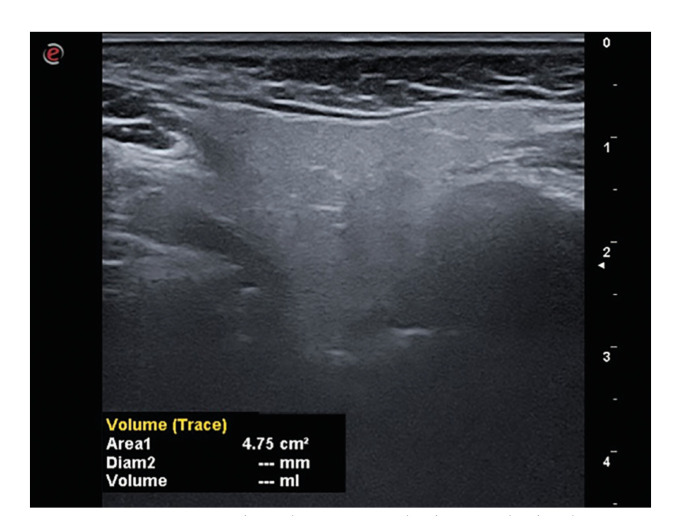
Transverse section ultrasonography image obtained to measure the volume of the parotid gland.
